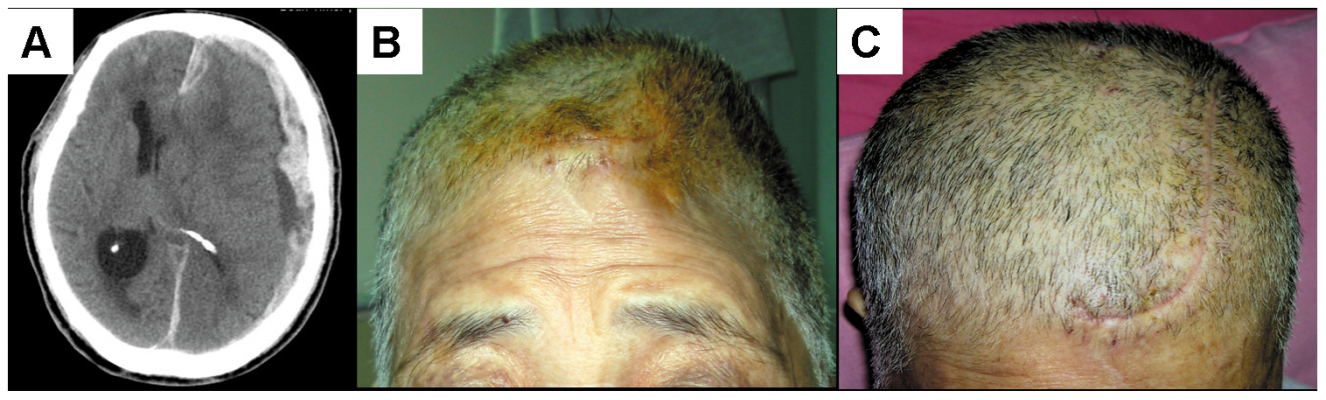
Representative photographs of case 14 before and after cranial implant reconstruction. (A). Initial CT scan of case 14, revealing acute subdural hemorrhage in the left temporal region with significant mass effect. (B). Patient's forehead appearance following DC due to TBI with acute subdural hematoma. (C). CAM-derived implant is based on regular 3-D CAD model in this case. The appearance of forehead following cranioplasty shows that reconstruction is symmetrical and stable after 20 months.
